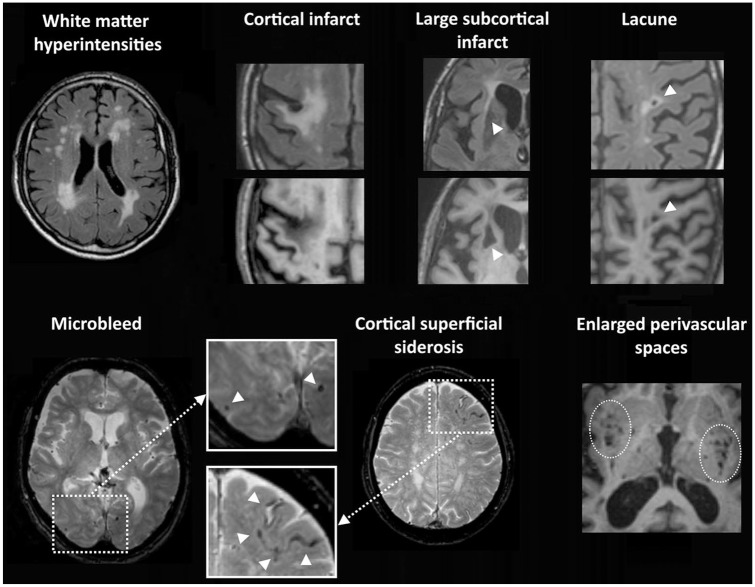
Vascular lesion types on brain MRI. Top row: white matter
